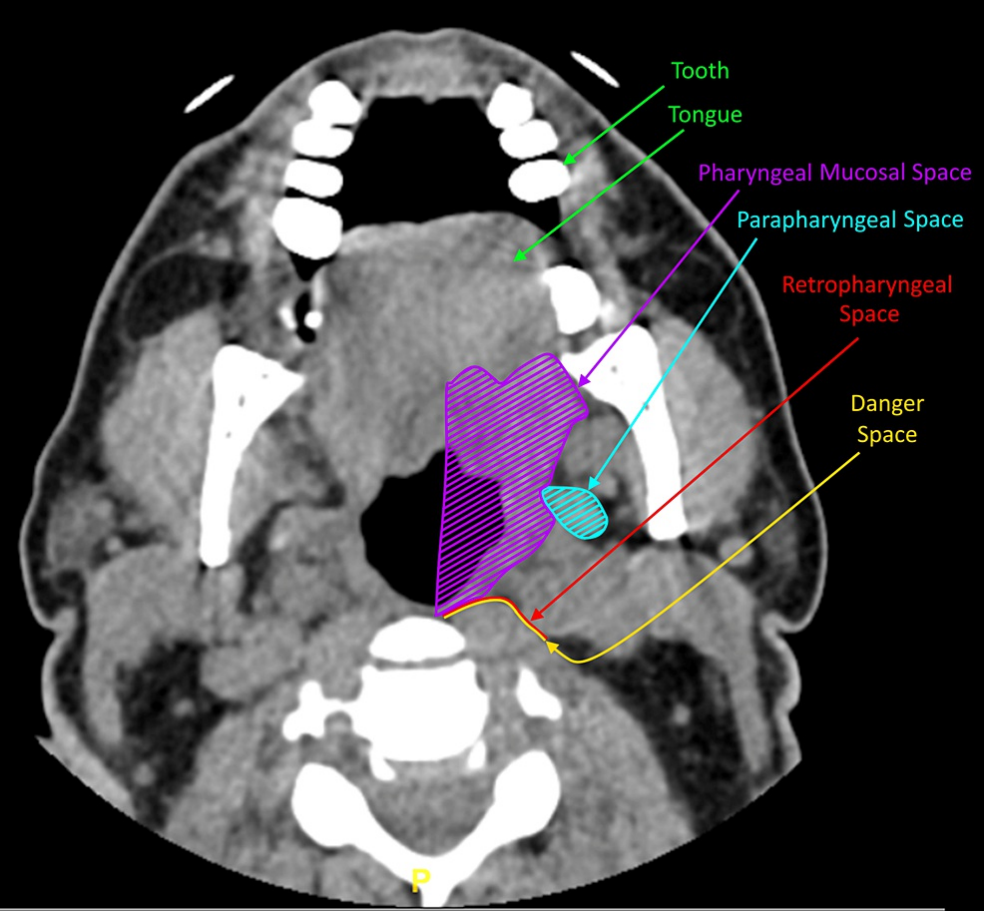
Transverse view of a CT scan with contrast of the patient's head Arrows indicate pertinent anatomical landmarks that infection may travel when spreading contiguously from the oral cavity into deeper tissue spaces.
